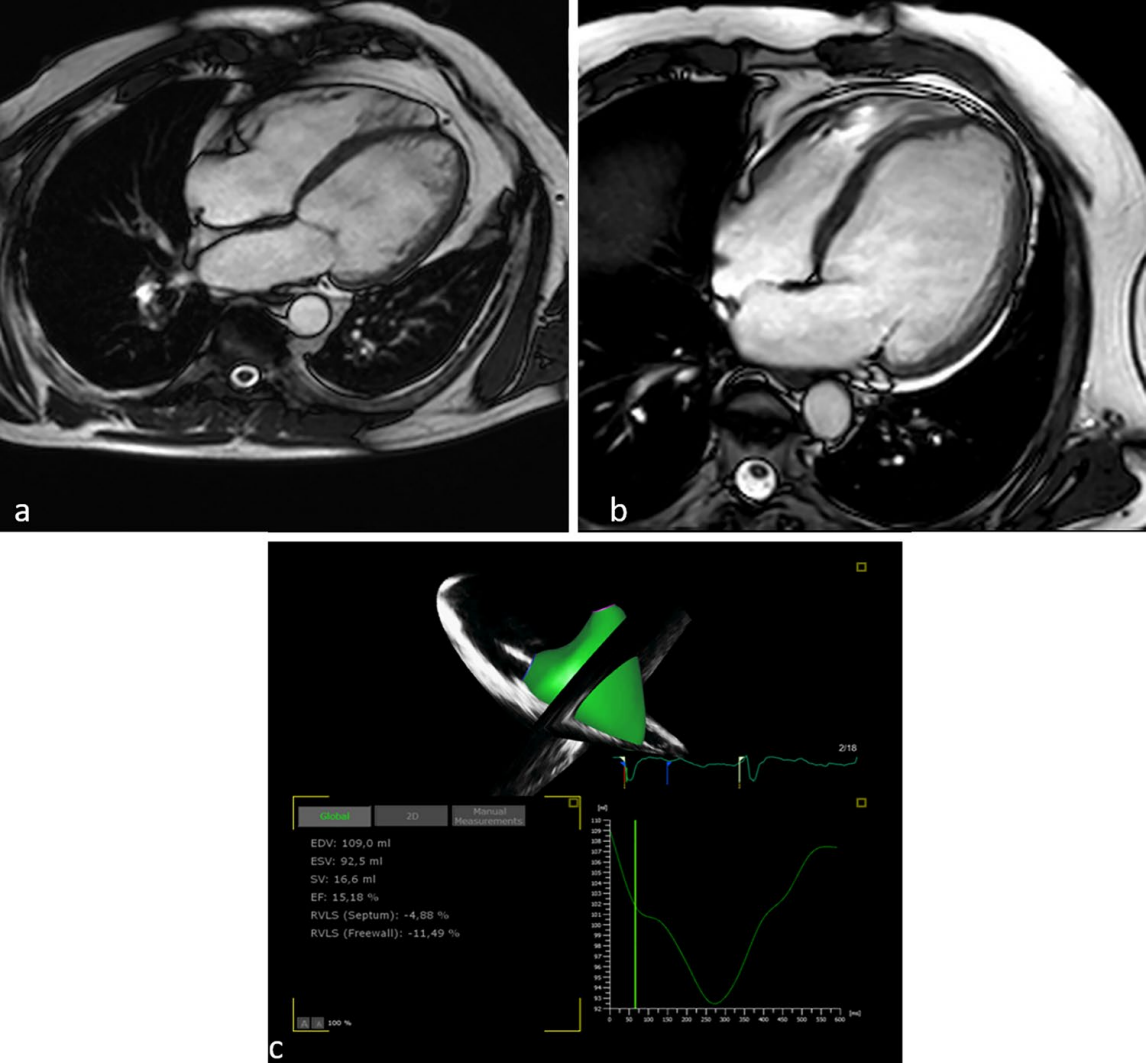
Cardiac magnetic resonance and 3D echocardiography evaluation of right ventricle. a A dilated right ventricle in a patient affected by dilated cardiomyopathy and biventricular dysfunction. b A dilated cardiomyopathy without involvement of right ventricle. c A severely depressed right ventricular function analyzed with 3D echocardiography
